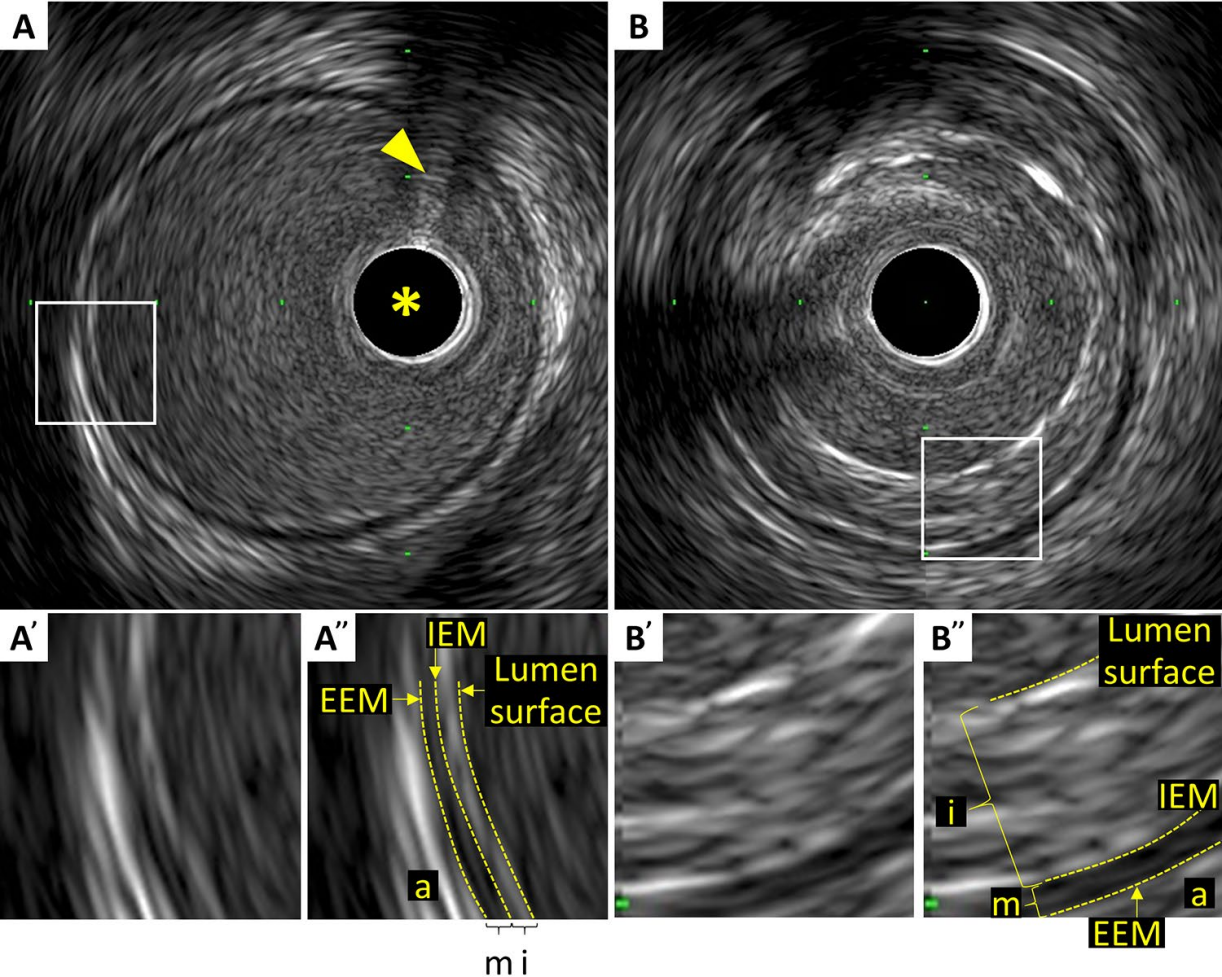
Three layers of coronary arteries on intravascular ultrasound. Coronary arteries consist of three layers (“i”—intima, “m”—media, “a”—adventitia). The innermost layer, tunica intima, comprises three elements: endothelium (corresponding to the lumen surface), atheroma (if the arteries are diseased), and internal elastic membrane (IEM). The second layer is tunica media, which consists of smooth muscle and external elastic membrane (EEM). The third and outer layer is tunica externa, which comprises the adventitia and peri-adventitial tissues. A Normal coronary artery. B Diseased vessel. Asterisk indicates intravascular ultrasound catheter, and arrowhead indicates guidewire with acoustic shadow
